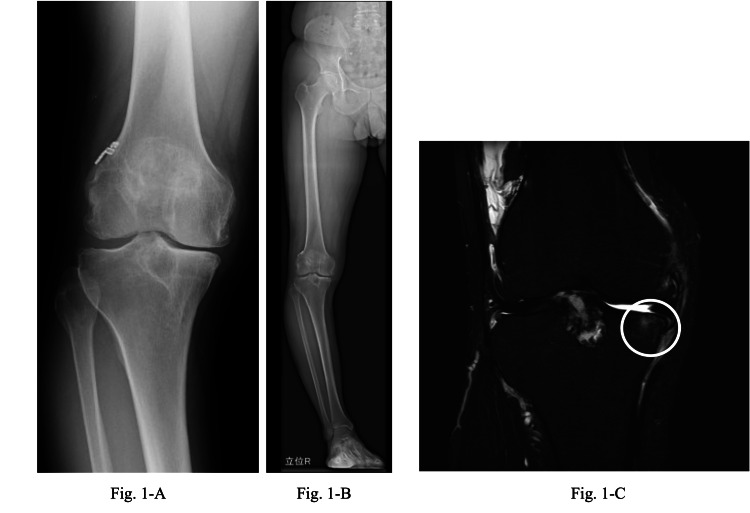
X-ray and MRI images of the patient before intervention. (A) X-ray: Medial knee OA (K-L grade 3; FTA: 187.5° in the right knee). (B) X-ray: %MA −13.1% in the right knee. (C) MRI: grade 2 BMLs were seen in the proximal medial part of the tibia. OA, osteoarthritis; MRI, magnetic resonance imaging; BML, bone marrow lesion; MA, mechanical axis; FTA, femorotibial angle; K-L, Kellgren-Lawrence
